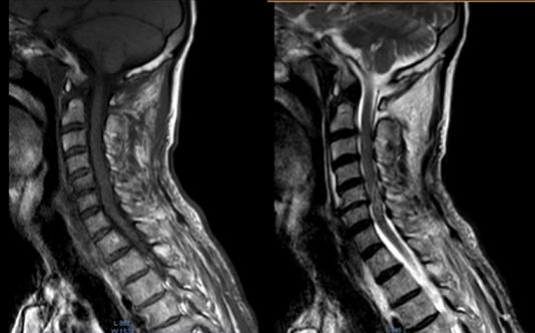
T1 and T2 weighted magnetic resonance imaging: Images show C4-C5 and C5-C6 degenerative disc disease and spinal cord swelling
